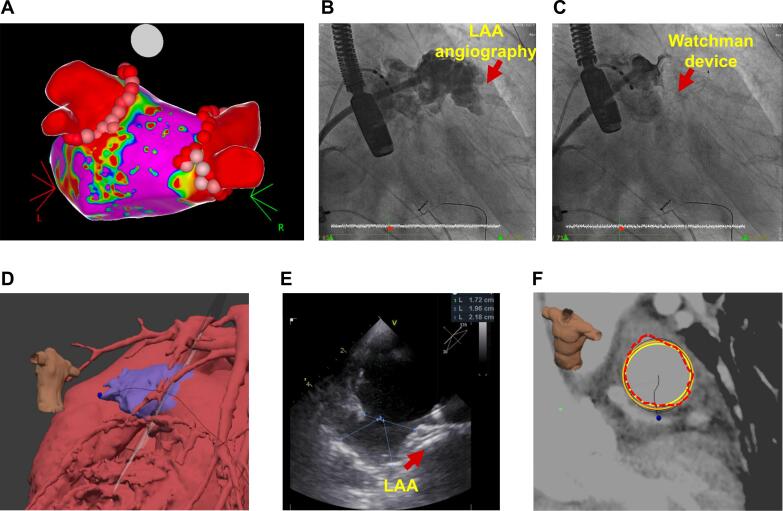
Representative case of radiofrequency ablation with concomitant LAAO In this representative case, we first performed an atrial septal puncture, followed by pulmonary vein isolation (PVi) using 3D mapping-guided radiofrequency ablation (A). This is followed by left atrial appendage (LAA) angiography (B) and implantation of an LAA occluder (C). Preprocedural CT planning images display various angles and automatically highlight the LAA (D). For comparison, intra-procedural transesophageal echocardiography (TEE) was performed (E). Analysis of the LAA ostium automatically calculated key anatomical data and provided recommendations for a suitable implant size (F).
